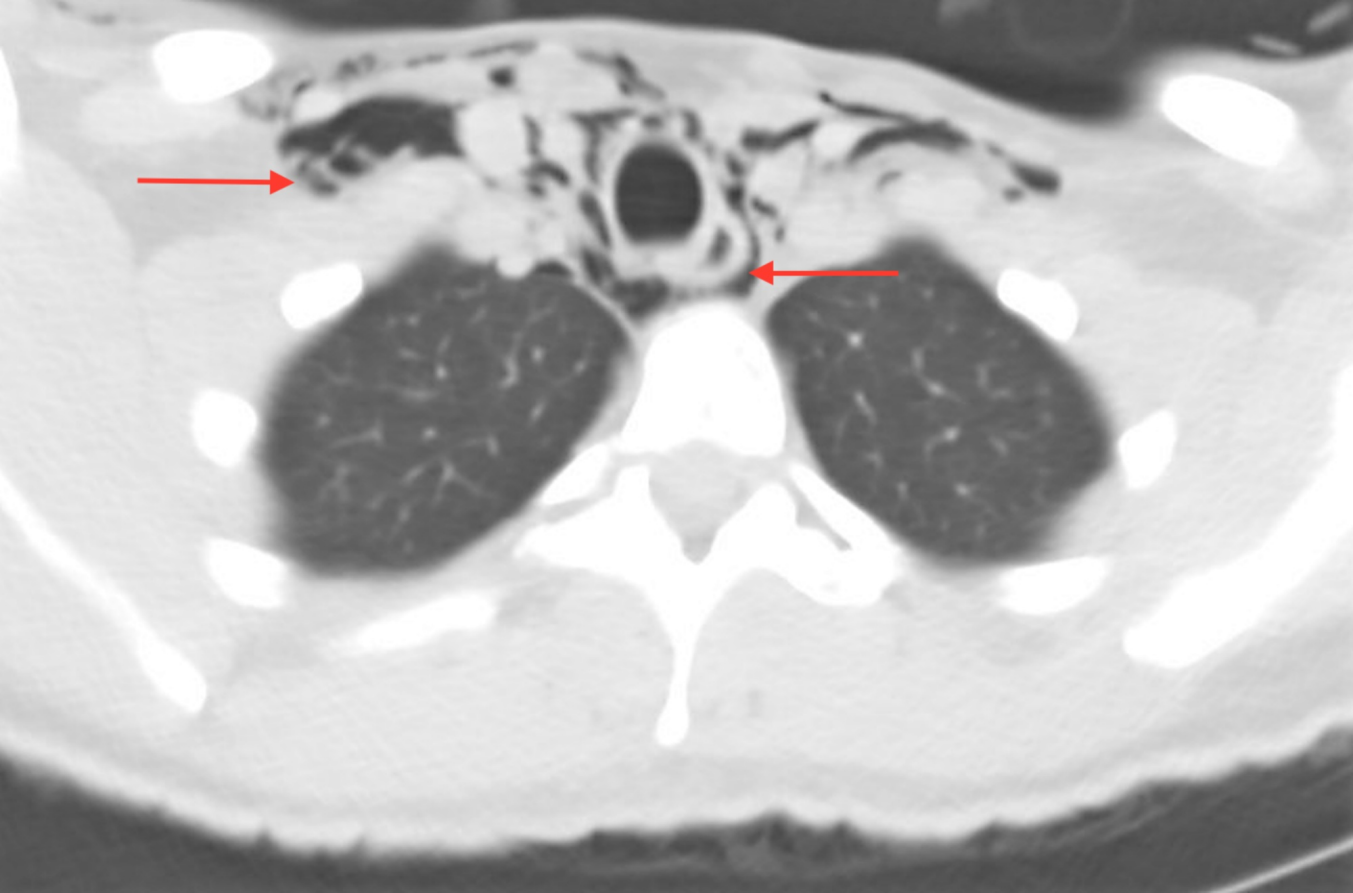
Axial CT image of the superior mediastinum.
